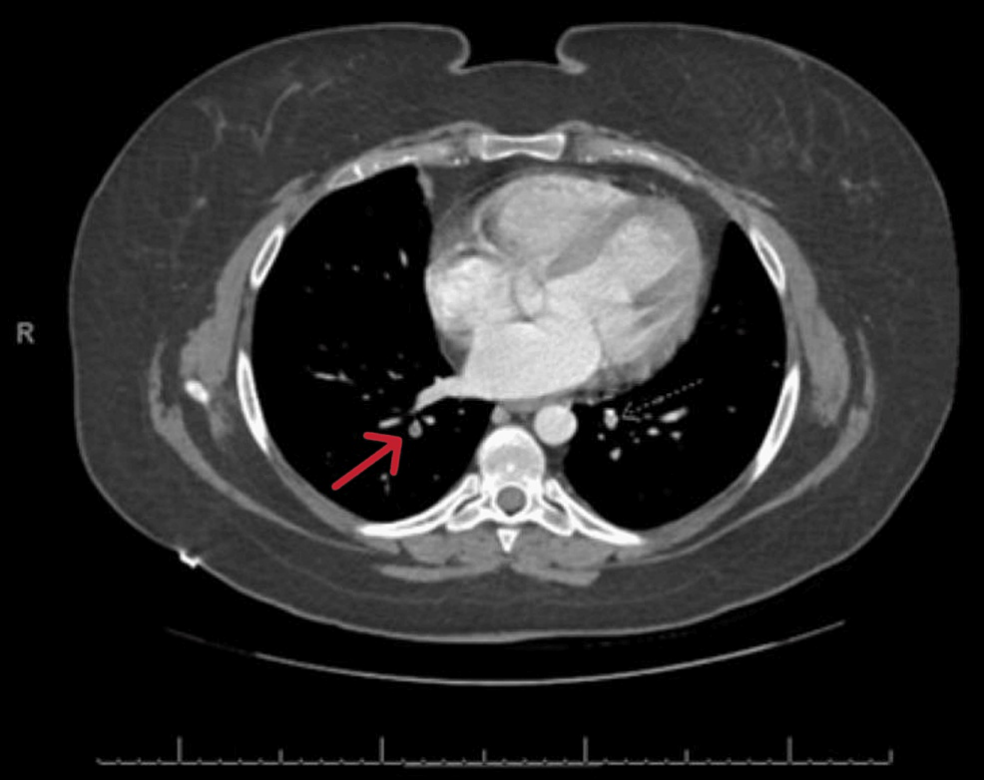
CT scan of the chest (axial view) with contrast showing the right pulmonary embolism (red arrow).
